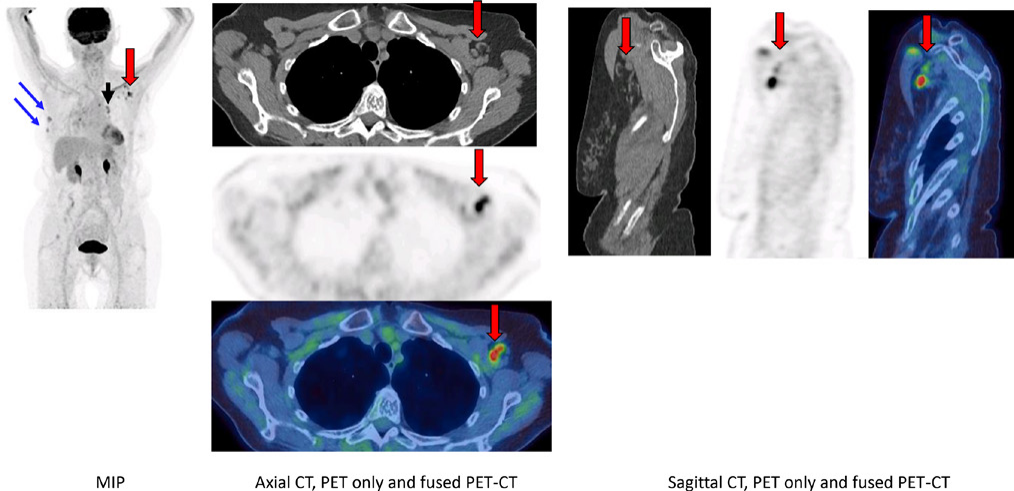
FDG PET-CT (MIP, axial PET only, fused PET-CT, CT only, sagittal PET only, fused PET-CT and CT only) demonstrated unchanged intense uptake in two stable right breast nodules (blue arrows), unchanged intense uptake in bilateral mediastinal and hilar lymph nodes (black arrows). Intense uptake in normal-sized left axillary and subpectoral lymph nodes with normal fatty hilum (red arrows).
